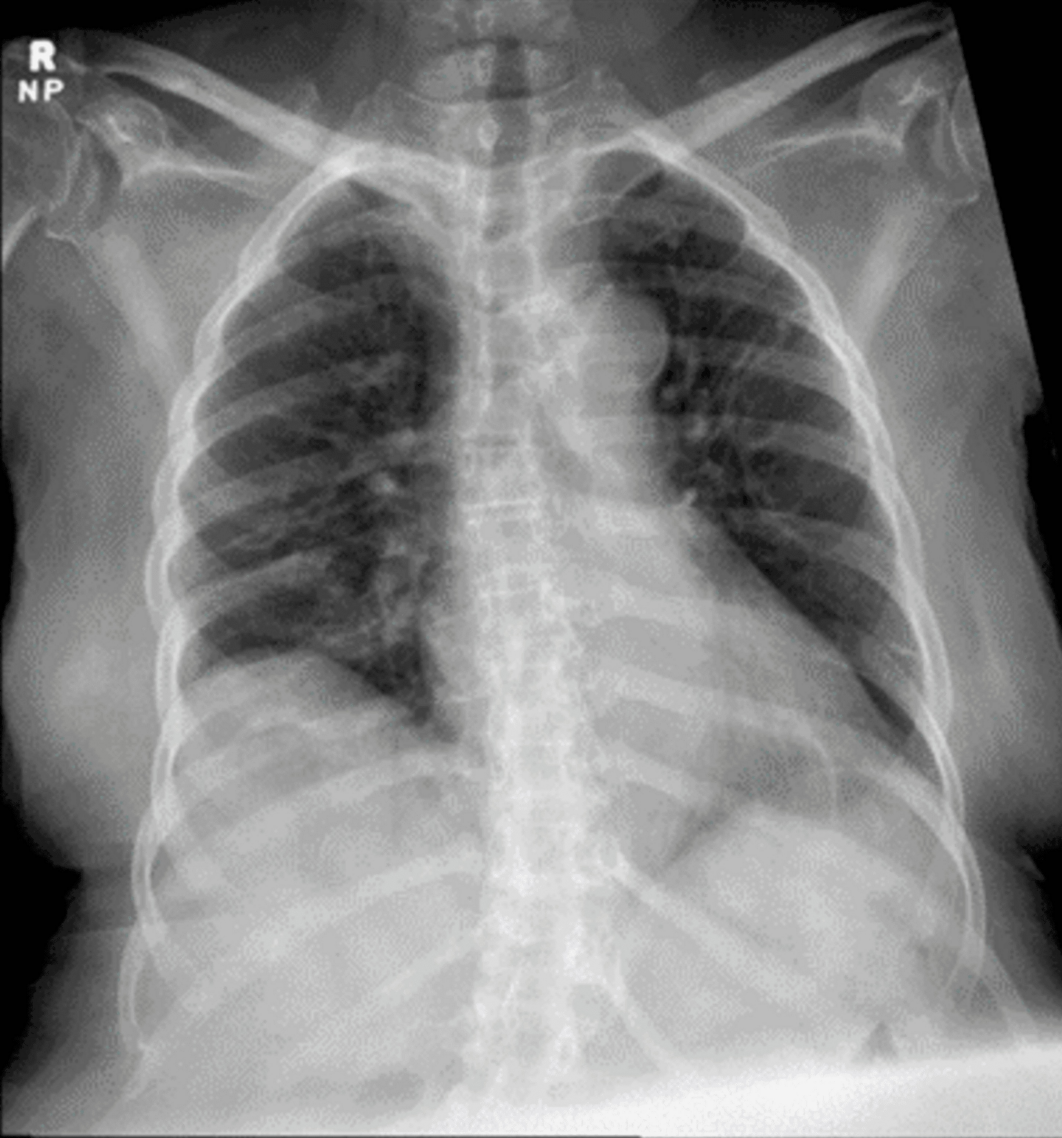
Anteroposterior CXR on the day of admission. CXR, chest x-ray
